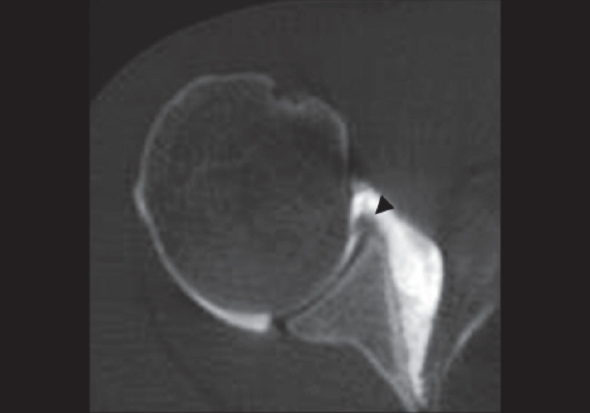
Axial CT-Arthrography image of the 27-yearold man with arthroscopy proved Bankart lesion. The CT-Arthrography image shows only the capsular tear, but no contrast media between the labrum and the glenoid rim is present (*arrow*). It was interpreted as normal labrum
